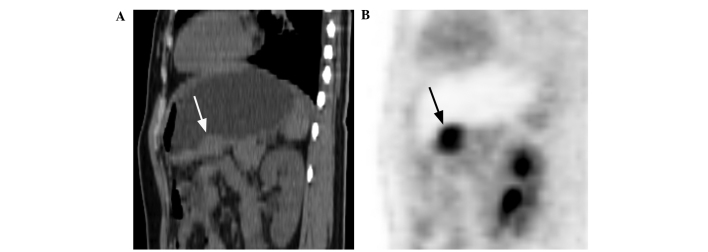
A 44-year-old female with gastric poorly-differentiated adenocarcinoma accompanied by partial signet ring cell carcinoma. (A) In the sagittal computed tomography image, local thickened gastric wall can be observed in the body of the stomach. (B) In the sagittal positron emission tomography image, local increased ^18^F-fluorodeoxyglucose accumulation (maximal standard uptake value, 5.6) can be confirmed in the corresponding gastric region.
